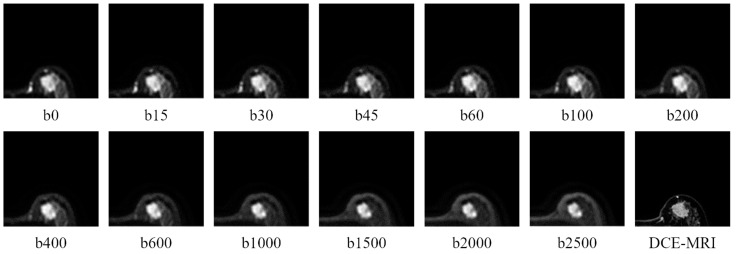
IVIM-DW images of the left breast with a tumor at different b-values (0~2500) and DCE-MR imaging of the left breast.
